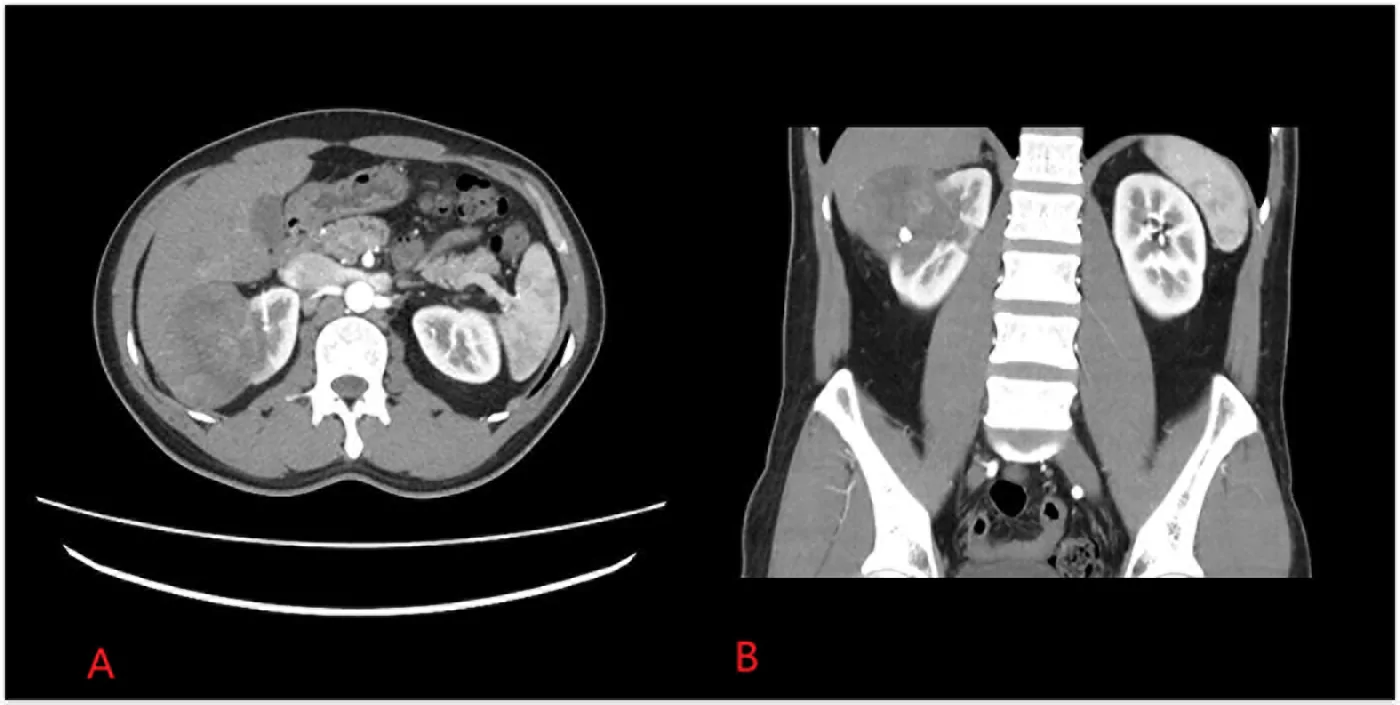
Abdominal computed tomography (CT) scan. Coronal (A) and axial (B) CT projections showed a large inhomogeneous mass measuring 72 × 65 × 66 mm in the right kidney tumor, showing a mixed density shadow, accompanied by nodular high-density lesions and patchy low-density areas, with clear boundaries, and delayed inhomogeneous enhancement after enhancement.
